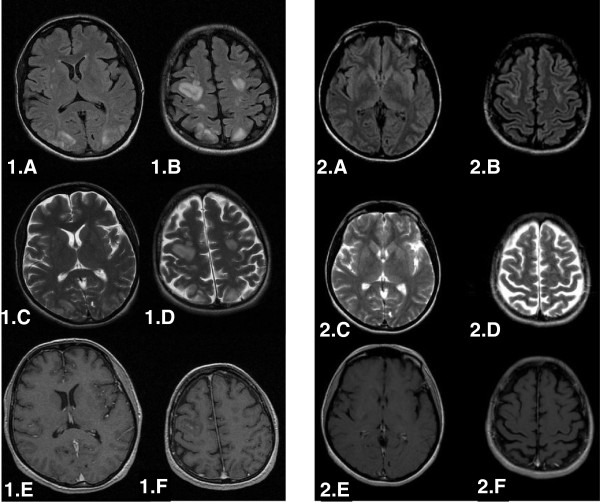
Brain MRI at the onset of the syndrome (1) and three weeks later (2).: Subcortical oedema at the occipital and parietal lobes bilaterally shown as hyperintense signals on FLAIR and T2 sequences, absence of lesions on T1 sequence. 1.A, 1.B (FLAIR), 1.C, 1.D (T2), 1.E, 1.F (T1), respectively. Three weeks later the subcortical oedema had been subsided from both FLAIR and T2 sequences. : 2.A, 2.B, 2.C, 2.D respectively.
